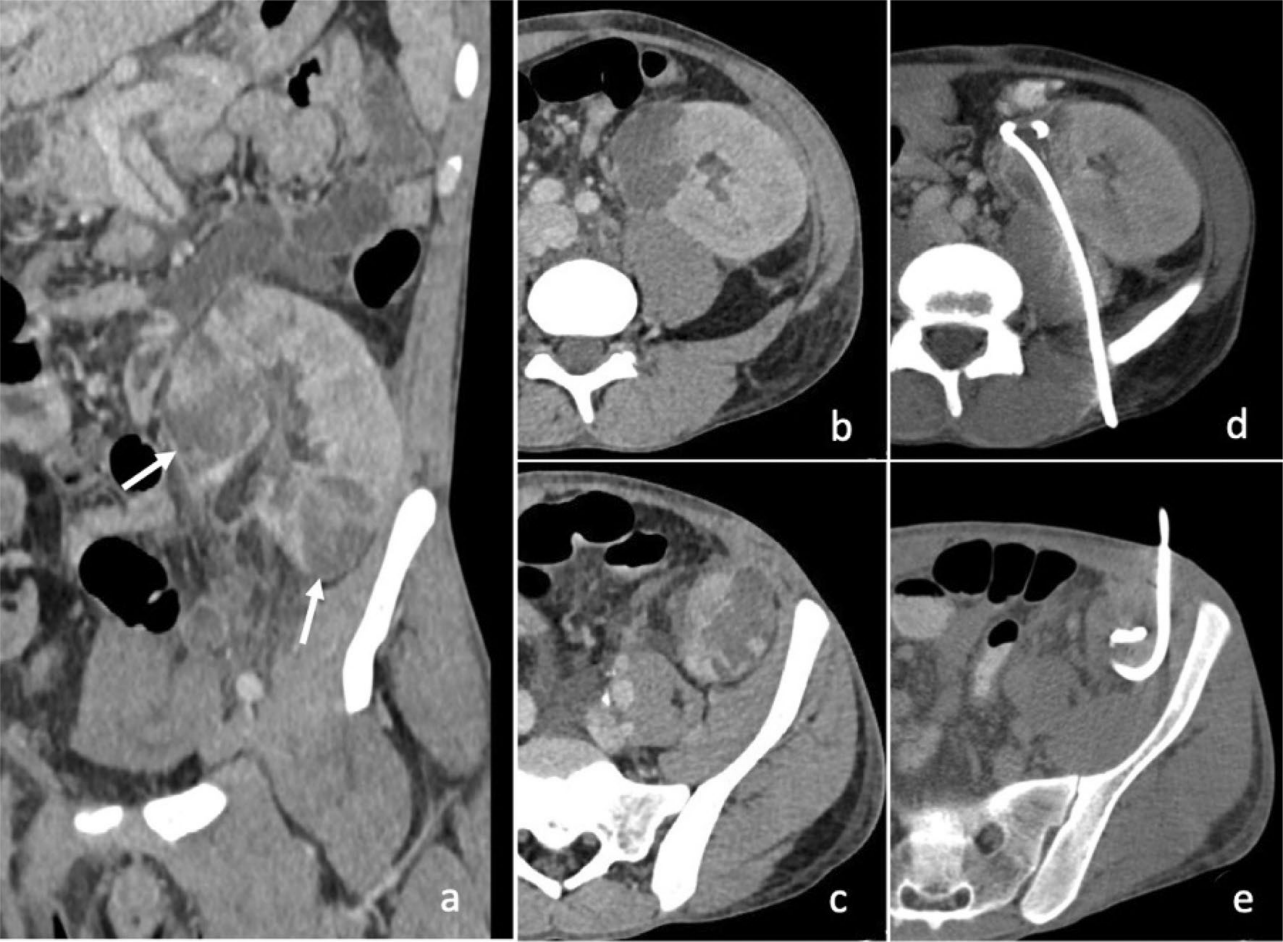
Coronal (a) and axial (b, c) enhanced CT scans of a 34-year-old patient with two abscesses in a transplanted kidney in the left iliac fossa (white arrows in a), drained with two 12F PIGTAIL catheters. The abscess of the upper pole of the kidney (b) required a posterior approach because of the interposed colon (d). The lower pole of the kidney was drained using an anterior approach (c, e)
